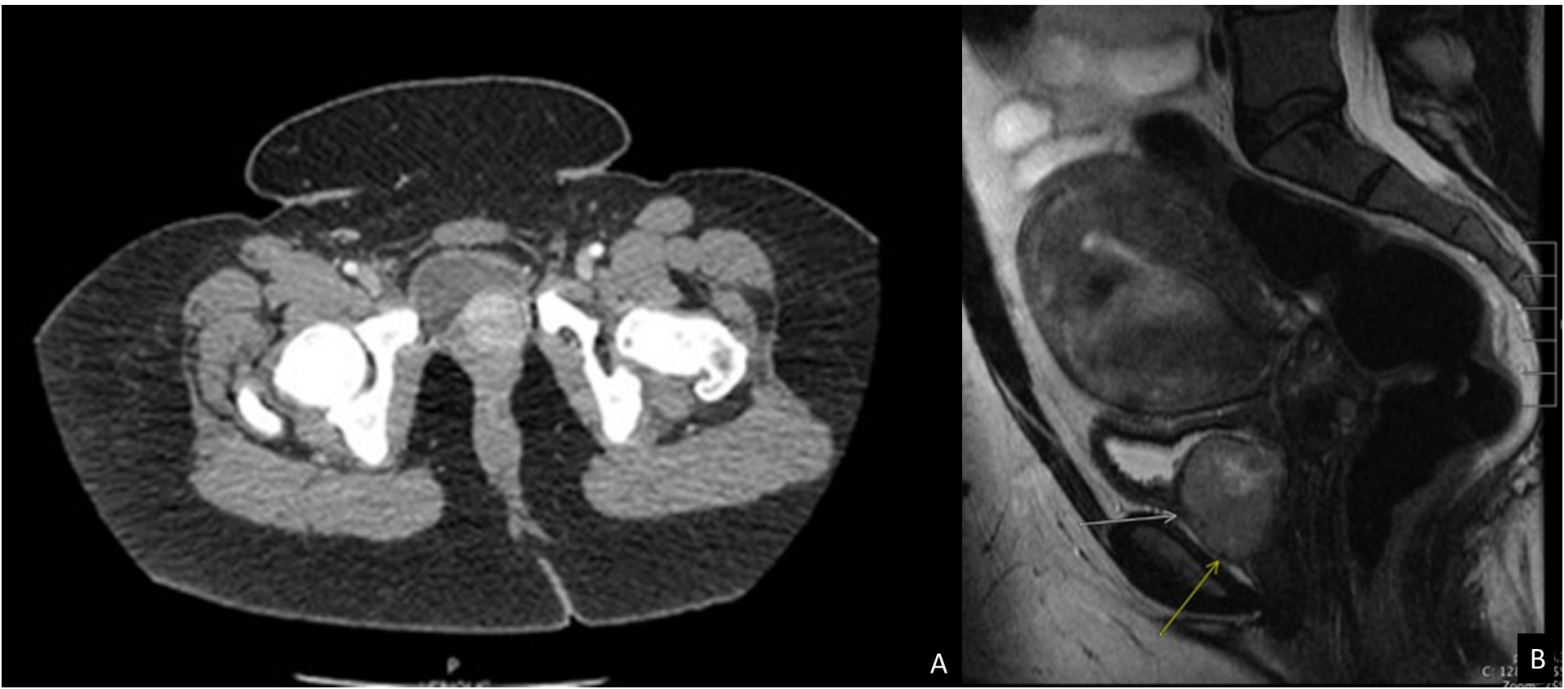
Imaging studies of the patient. (A) CT Intravenous pyelogram demonstrating an enhancing 3.7 cm bladder mass centered near the left ureterovesical junction without hydronephrosis or lymphadenopathy. (B) MRI pelvis and abdomen showing a well-defined 3.8 cm mass (arrows) in the anterior inferior aspect of the bladder abutting the urethra and vagina with a mild hypertense signal on T2 weighted image.
